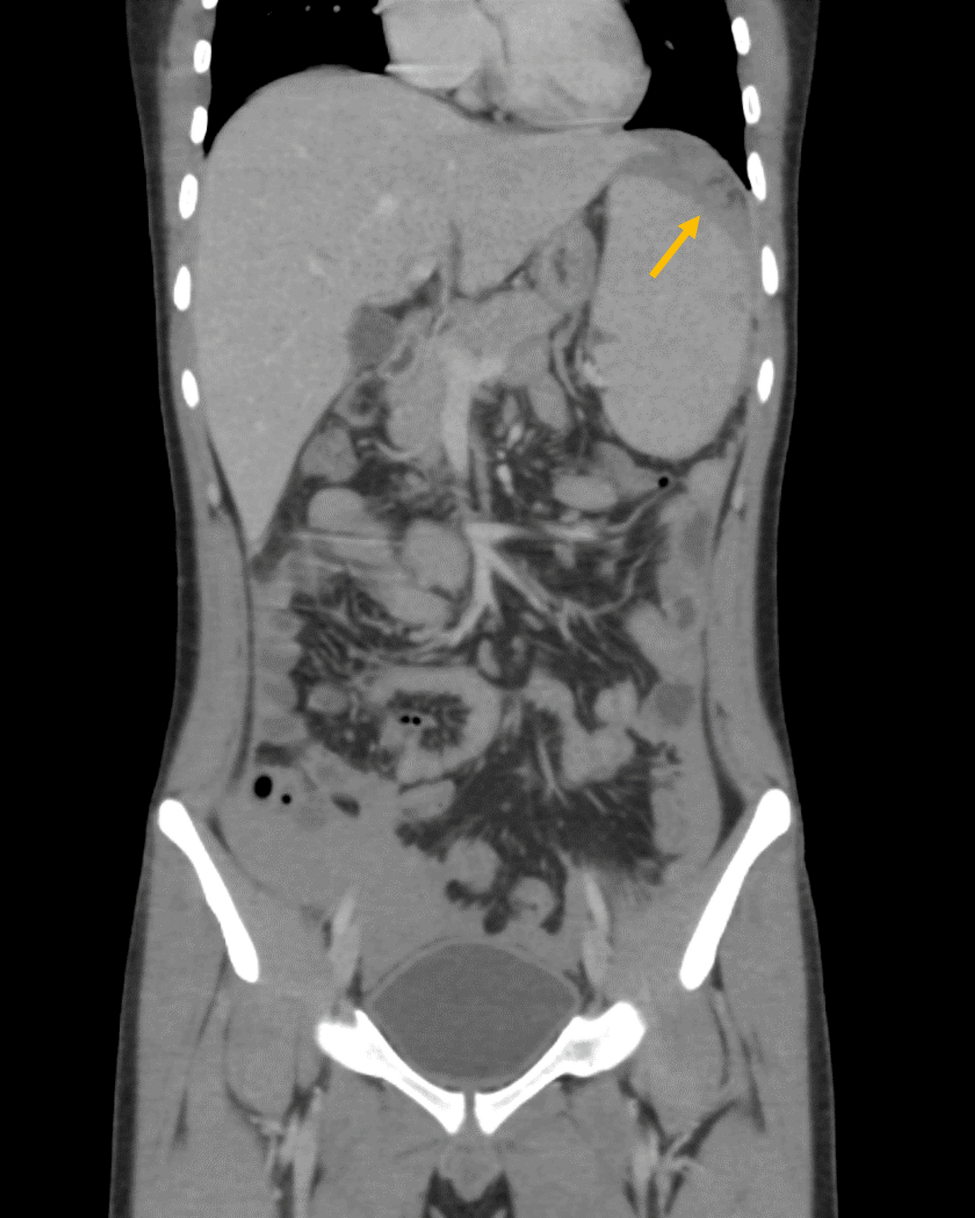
Abdominal CT scan (portal venous phase) with an IV contrast coronal cut demonstrating a mildly enlarged spleen with at least two small peripherally located splenic lacerations (arrow) measuring approximately up to 1 cm
